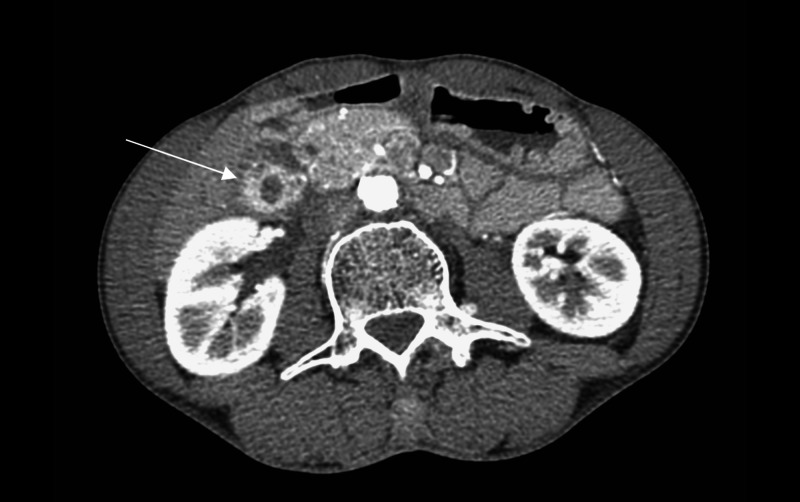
Axial contrast-enhanced CT image shows concentric thickening and enhancement of the gallbladder wall at the body and fundus with small mural cystic foci (arrow) representing the “rosary sign”.
